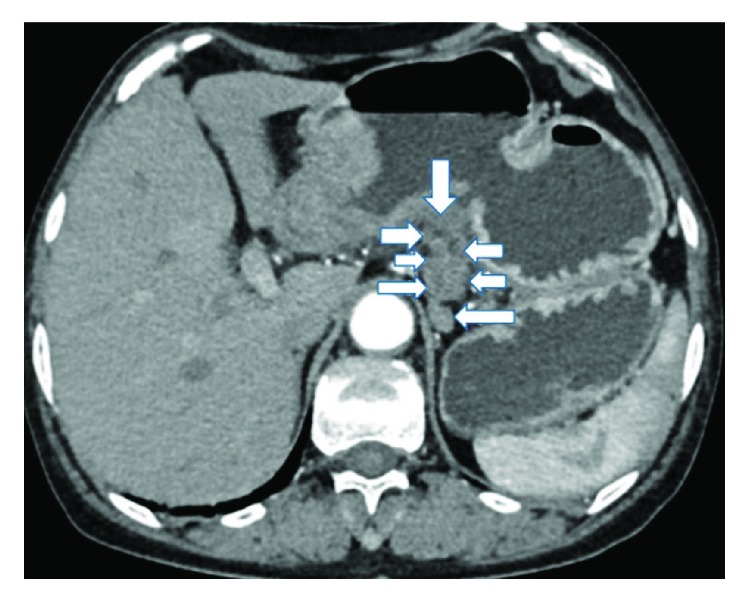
CT image obtained in a 65-year-old woman. Clustered lymph nodes (>6) can be seen along the lesser curvature of the stomach.
